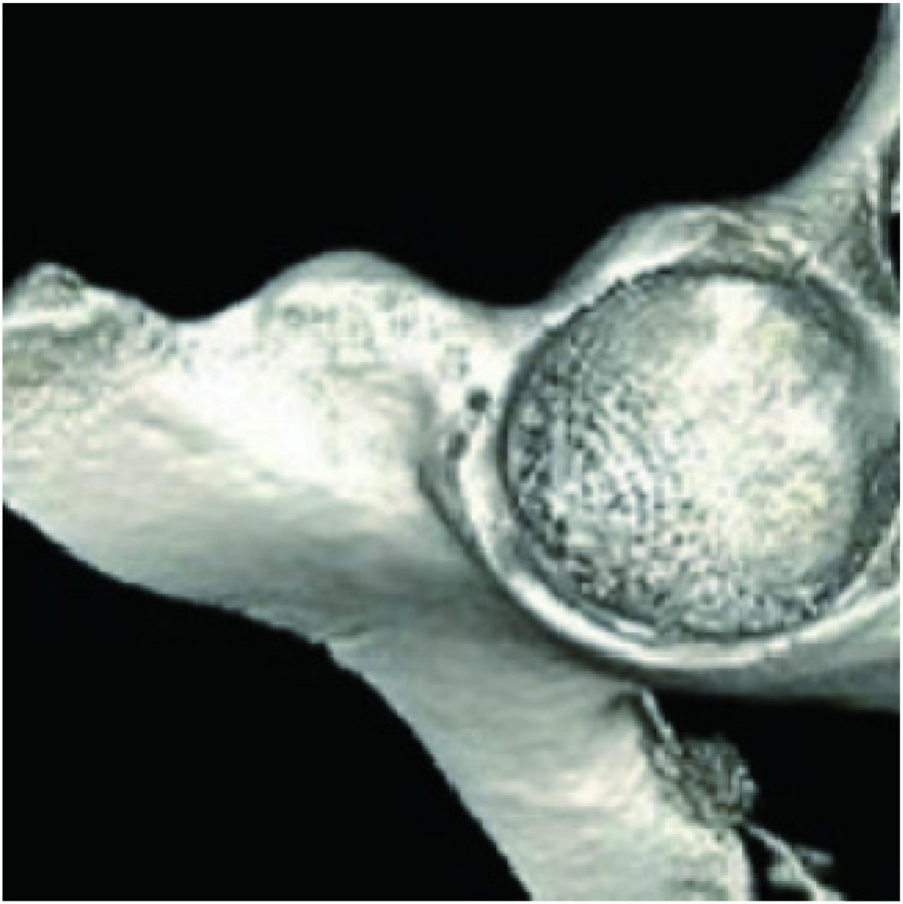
Postoperative three-dimensional CT.
